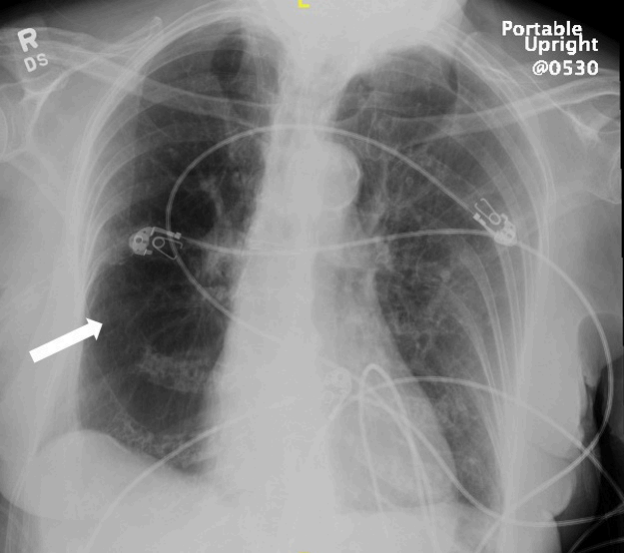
Chest radiograph. Image is notable for increased lucency within the right hemithorax (arrow).
